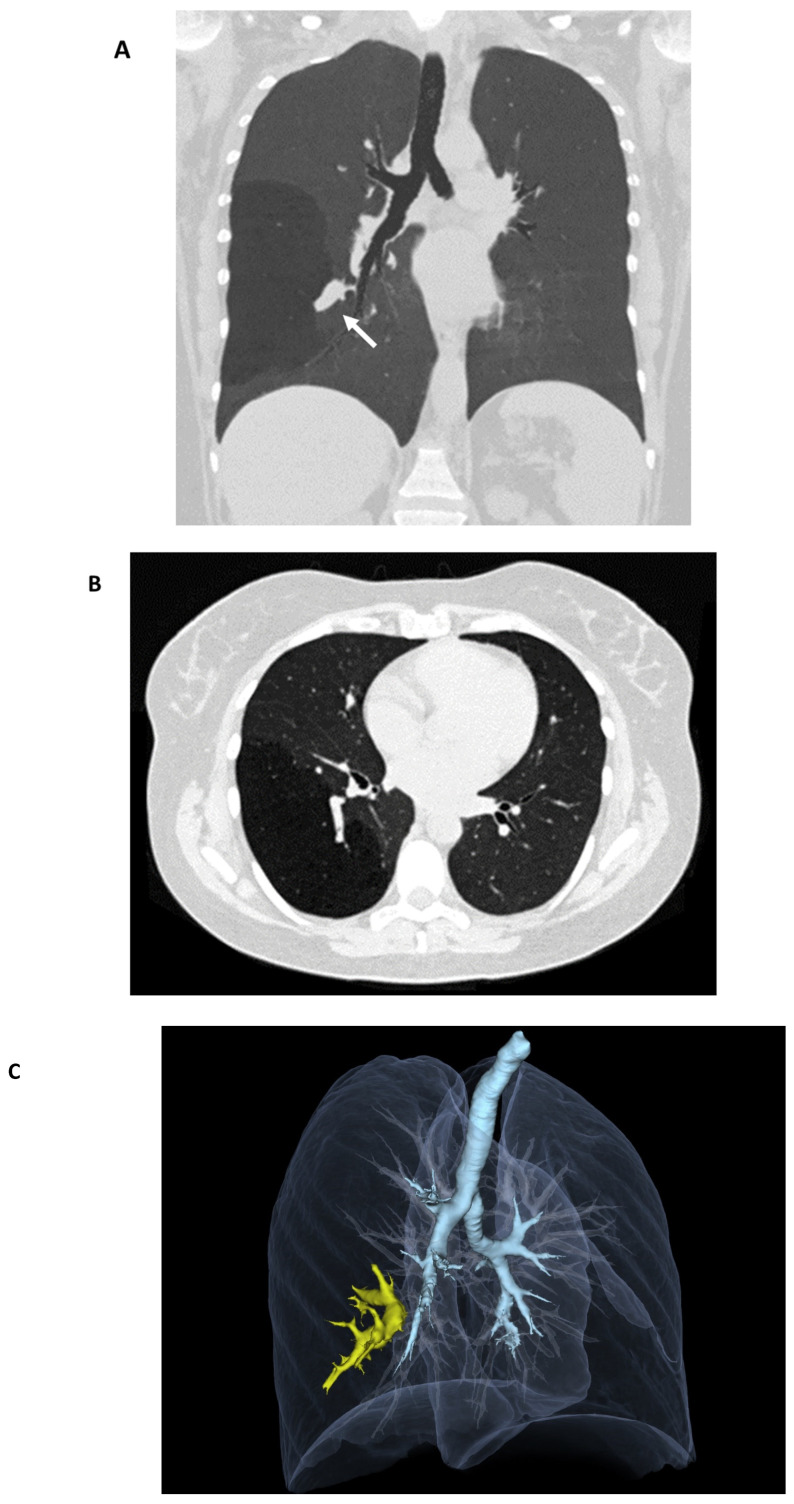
Bronchial atresia (BA) is a rare congenital disorder and usually evolves as a consequence of a bronchial artery occlusion or an intrauterine infection during the prenatal period [1]. Typically, segmental bronchi are affected. Distal to the atresia, a mucocele can develop. As a consequence, the lung parenchyma distal to the affected bronchus is hyperinflated. This is caused by collateral ventilation through intra-alveolar pores of Kohn. BA is typically found in the upper lobes [2,3]. (A,B) The asymptomatic patient presented here had a computed tomography (CT) which revealed a well-circumscribed emphysema of the right lower lobe as an incidental finding. (A,B) shows a complete obstruction of the right lateral-basal segmental bronchus (B9). The proximal part of the segmental bronchus is obliterated while the distal part is dilated and filled with fluid consistent with a bronchocele ((A), white arrow). (C) Three-dimensional volume-rendered CT reconstruction of the tracheobronchial tree and lungs depicts the patent central and segmental bronchi in light blue, while the isolated mucus-filled distal B9 bronchus, the bronchocele, is highlighted in yellow. The semi-transparent lung overlay provides anatomic orientation. At the expected origin of the right lower-lobe B9 segmental bronchus, the continuity with the proximal bronchial tree is missing, demonstrating the bronchial interruption which is characteristic for bronchial atresia. Surgical treatment of BA is only required if the patient becomes symptomatic. However, a follow-up CT scan is recommended to assess for progression of the hyperinflation.
